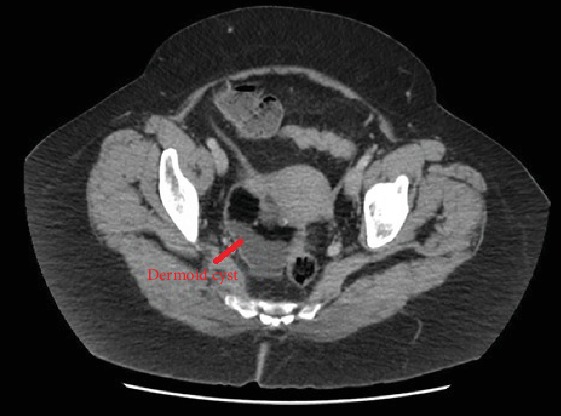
Transverse view of CT abdo-pelvis showing a dermoid cyst in the right adnexa.
